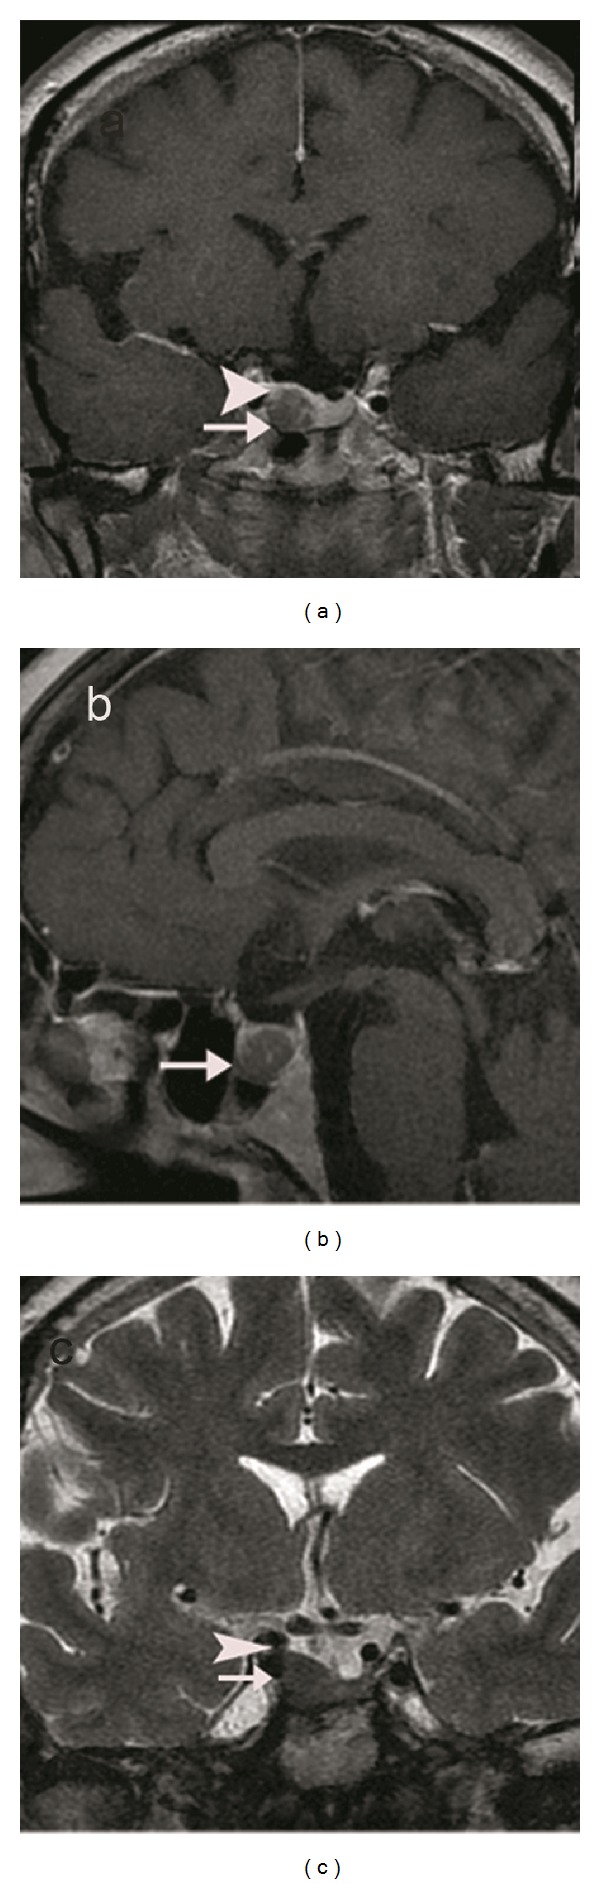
T1-weighted coronal (a) and sagittal (b) images enhanced with IV-GBCM and T2-weighted coronal (c) images are showing a hyperintense macroadenoma. Convexity of the right side of the gland (arrow heads) and the invasion of the right cavernous sinus (arrows) can be demonstrated on both T1-weighted and T2-weighted images.
